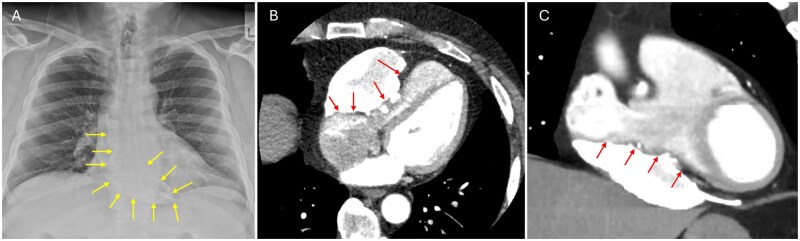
Preoperative Imaging. (A) Chest radiograph showing pericardial calcification (arrow). (B) Axial and (C) coronal computed tomography angiography demonstrating a calcified pericardial mass (arrows).
